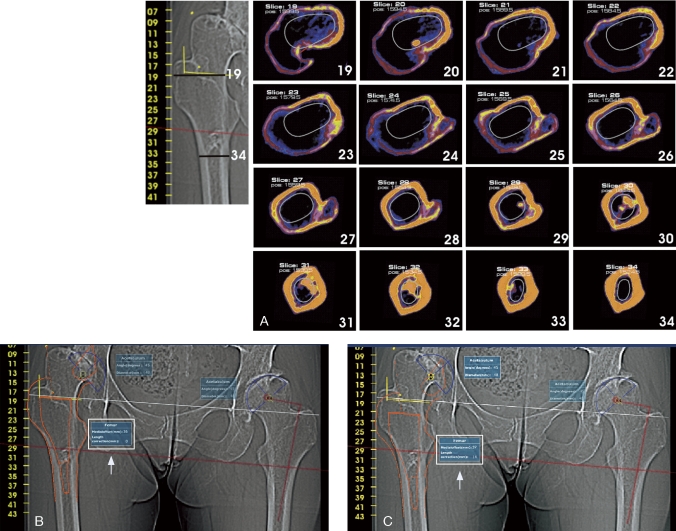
A. CT scans of the proximal femur, with 5-mm distance between each section. B. Digital template of the suggested stem design. C. Use of the template for determination of ideal medial femoral head offset and leg length. Information about medial femoral offset and leg length correction is automatically shown during use of the template. (The arrows show the text box where this information is given).
